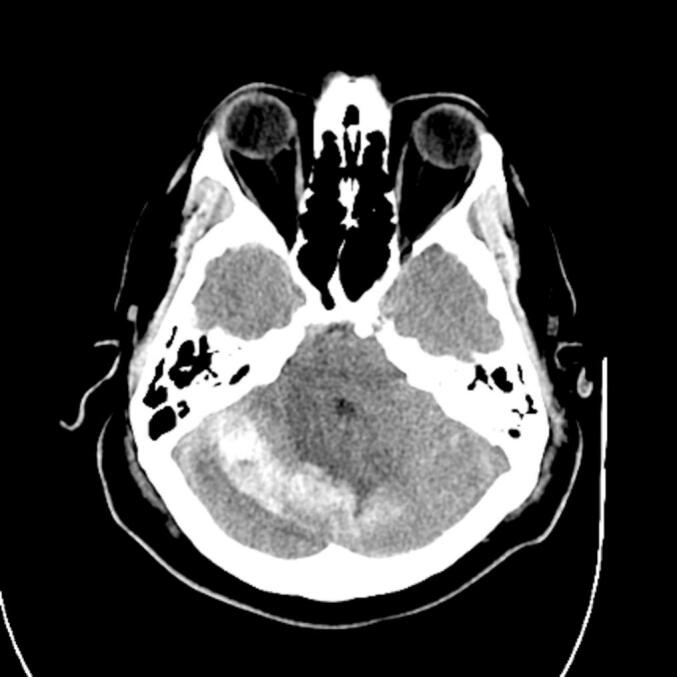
Head CT without IV contrast POD5 demonstrating a 1.7 cm × 4.9 cm × 1.5 cm right cerebellar hemorrhage with mild mass effect on the fourth ventricle.
